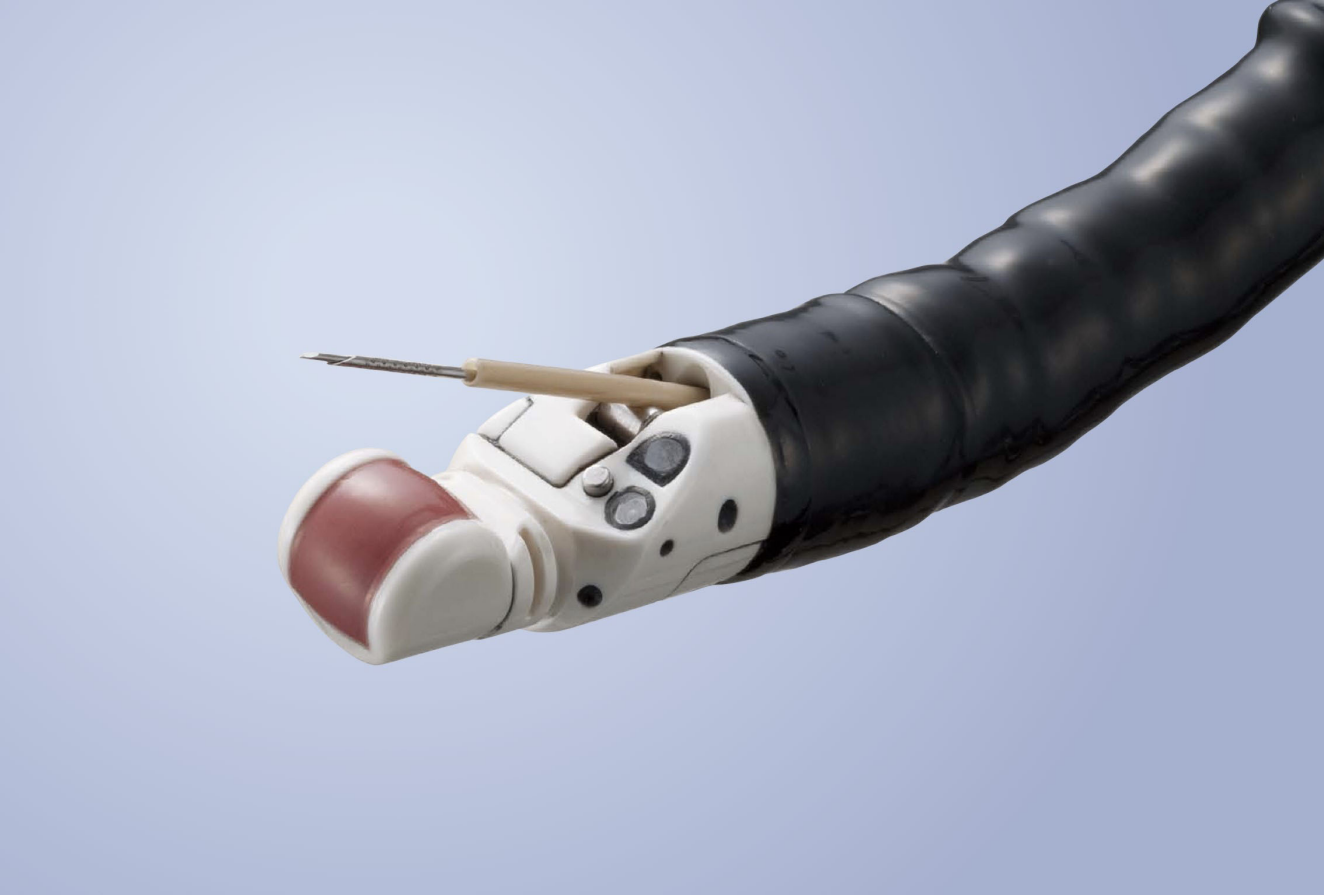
Linear Scanning Ultrasound Endoscope (GF-UCT180).
